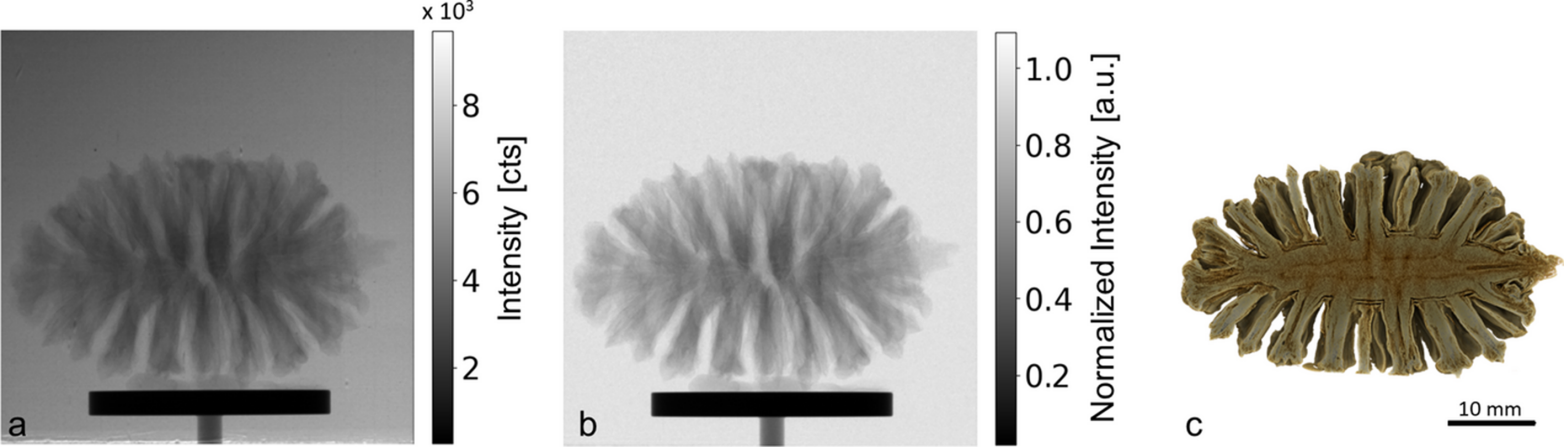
Tomographic measurement of a full pinecone with monochromated beam at 30.5 keV. The FOV of 5 cm × 5 cm (H × V) has been illuminated by the beam magnified by means of a Bragg-magnifier conditioner, achieving a flux corresponding to ∼500 X-ray photons pixel^−1^ s^−1^ (49.5 µm pixel size). The scale bar is equal in all of the images. (*a*) Projection image as recorded by the detector. (*b*) Flat-field-corrected image. (*c*) Cut in the centre volume rendering of the 3D tomography reconstruction obtained using *Drishti* (Limaye, 2012 ▸).
